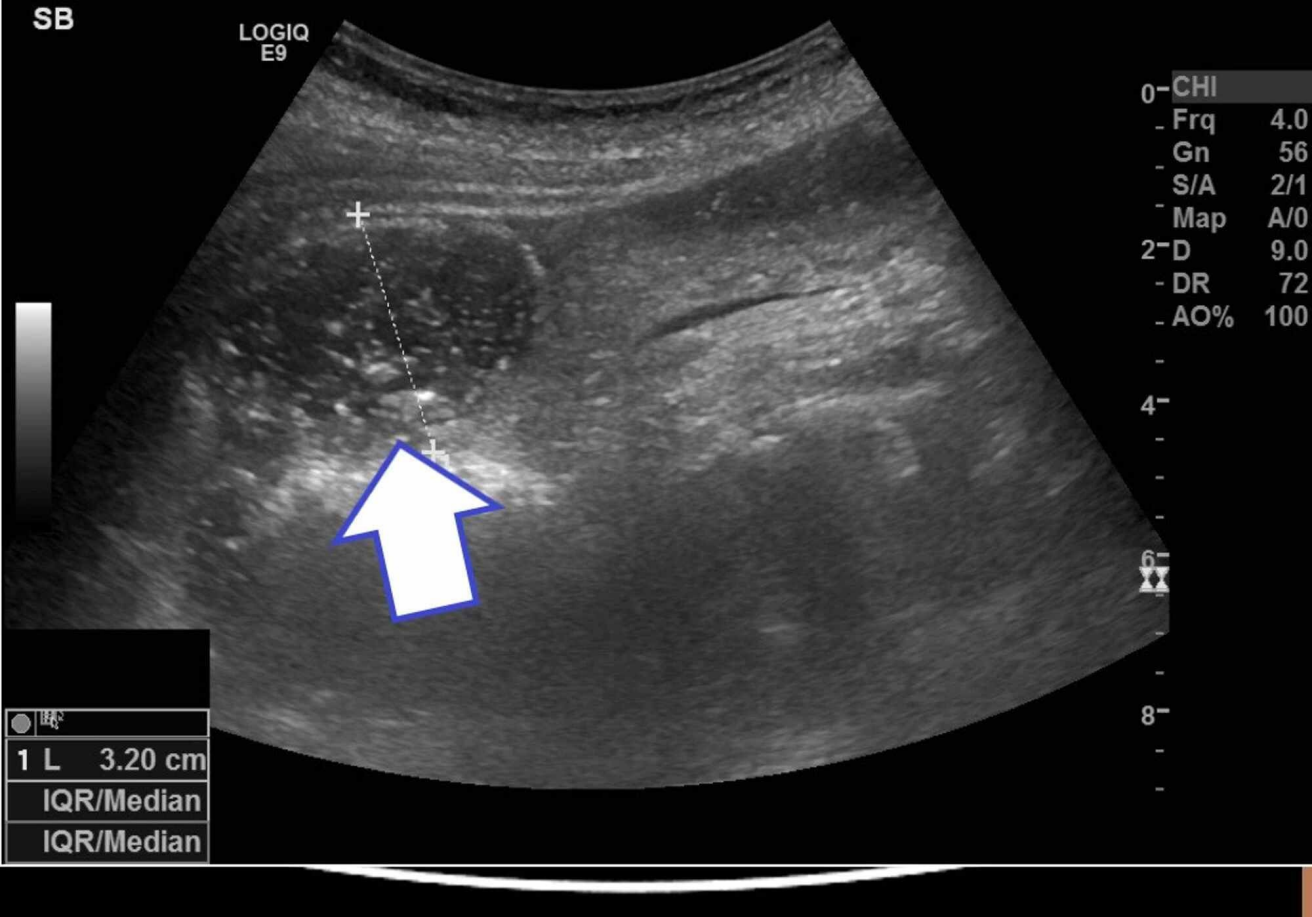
An ultrasound scan showing small bowel distension measuring 3.2 cm (white arrow indicates the distended small bowel)
